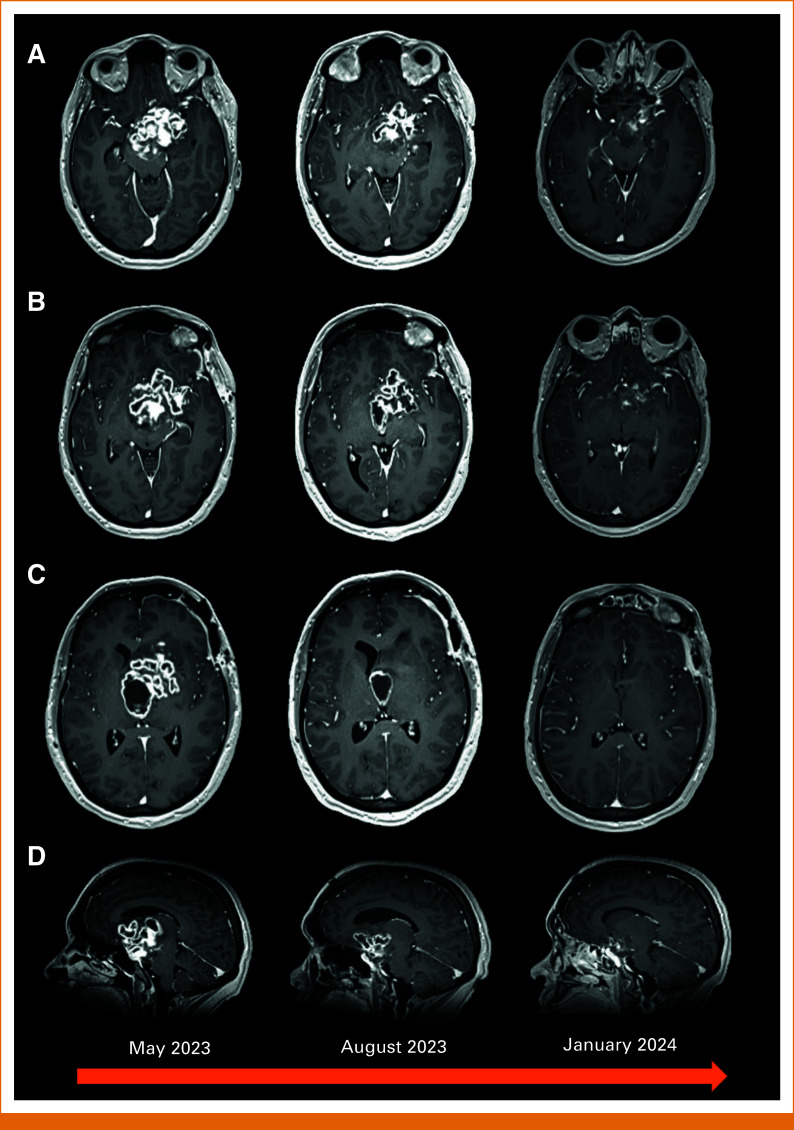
Magnetic resonance imaging of the brain: (A) Axial postcontrast at the level of the midbrain, (B) at the level of the hypothalamus, (C) at the level of the third ventricle, and (D) sagittal postcontrast. From left to right, the images show the treatment response as depicted in the significant reduction in size of the irregularly enhancing and multilobulated cystic suprasellar mass after 6 months of trametinib.
